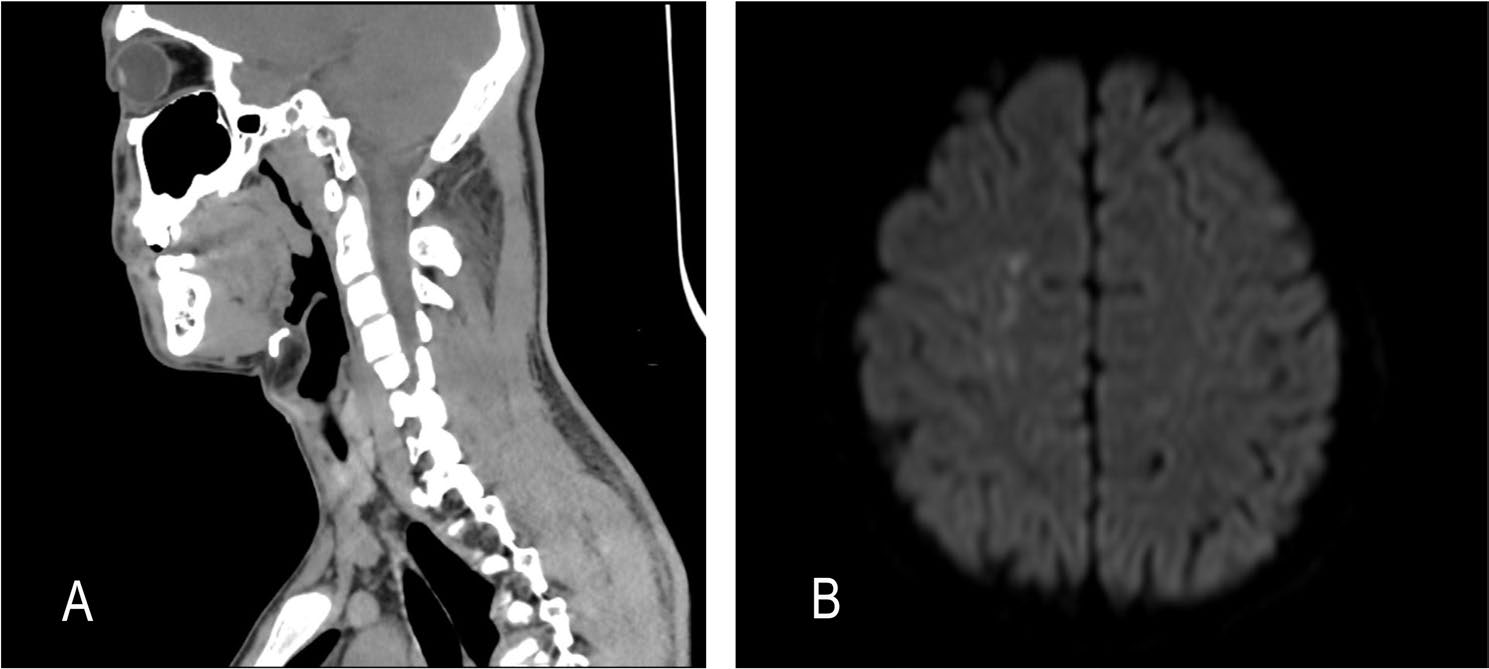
Neck CT revealed swelling of the left neck soft tissues, subcutaneous exudation, fluid accumulation, and mild swelling of the left parotid gland (A); Brain MRI upon admission showed patchy abnormal signal intensity in the right semioval center (B)
